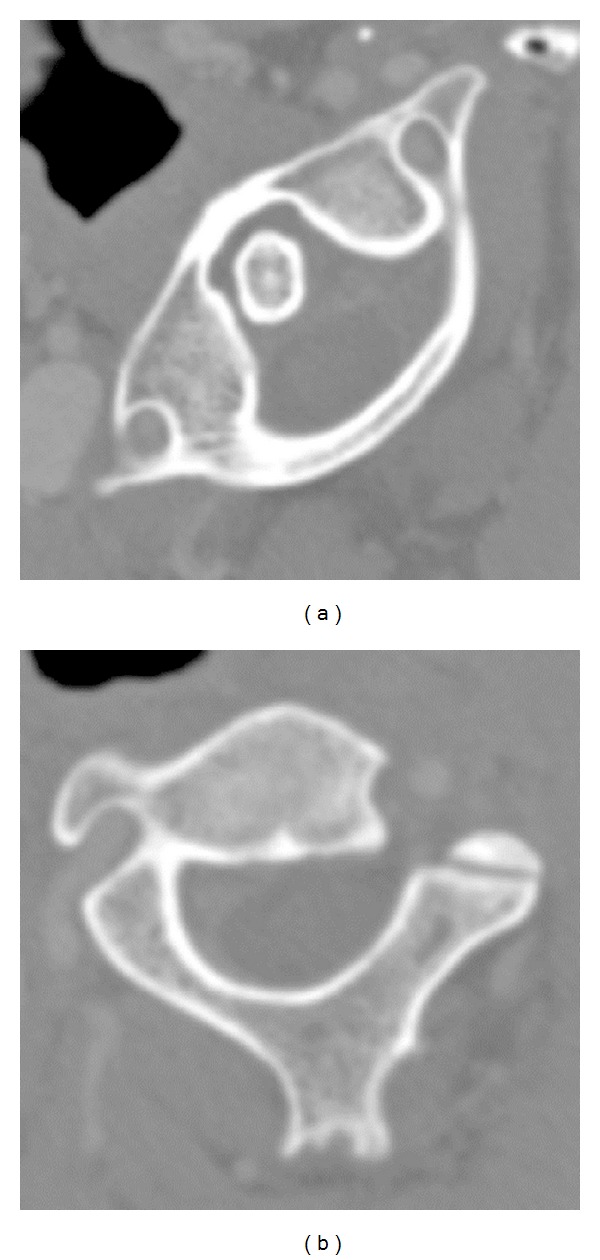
Computed tomography revealed that the atlas was rotated to the right centering on the dens of the axis. (a) Axial view of atlas and (b) axial view of axis.
